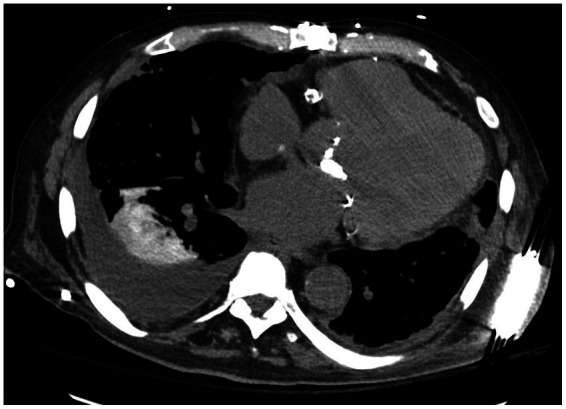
HRCT findings in amiodarone pulmonary toxicity. Lower lobe bilateral basilar infiltrates and pleural effusions especially on the right lung.
